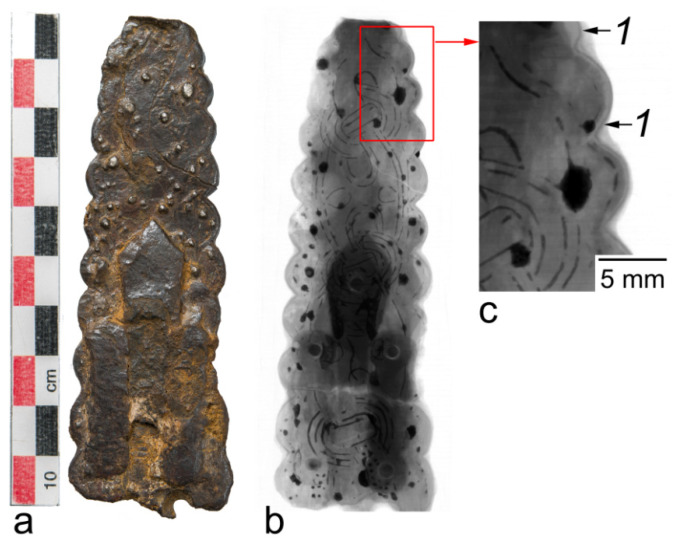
Study of the artifact from Chernaya Mogila: (a) photograph of the object; (b) SR radiograph; (c) enlarged part of the radiograph; 1—dense layer of mill scale.
